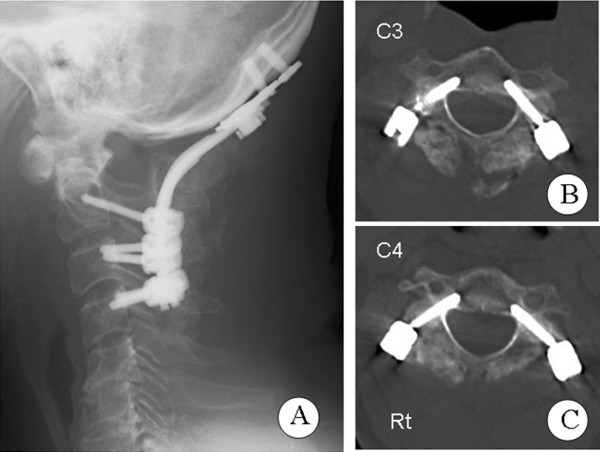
Postoperative images. (A) Lateral radiographs of the cervical spine and computed tomography images of the (B) C3 and (C) C4 vertebrae.
